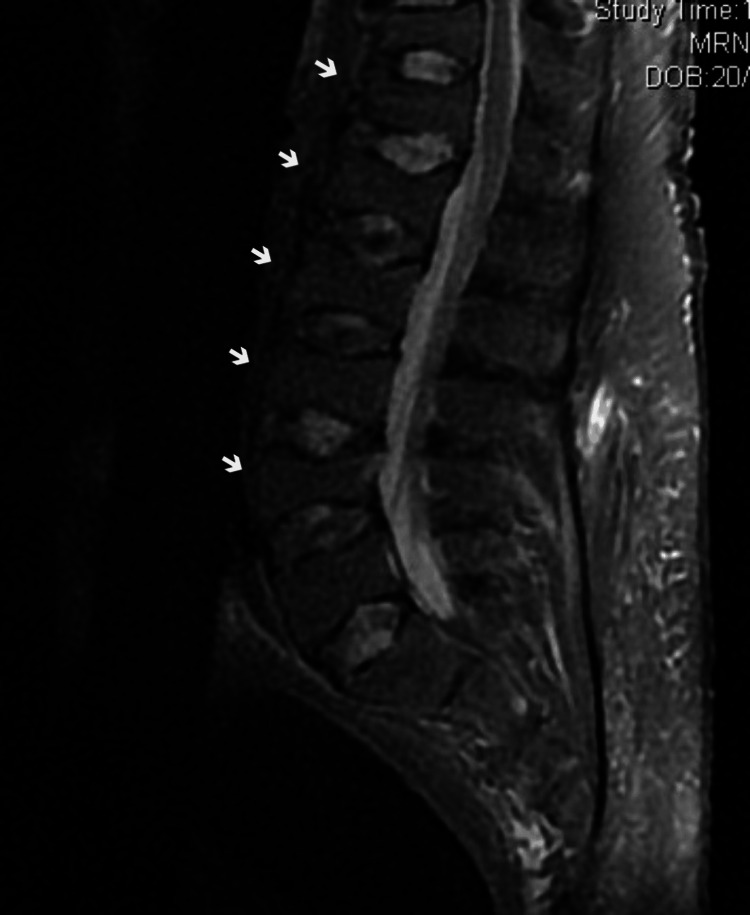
Sagittal view of dorsolumbar magnetic resonance imaging Arrows: compressive pathological fractures
